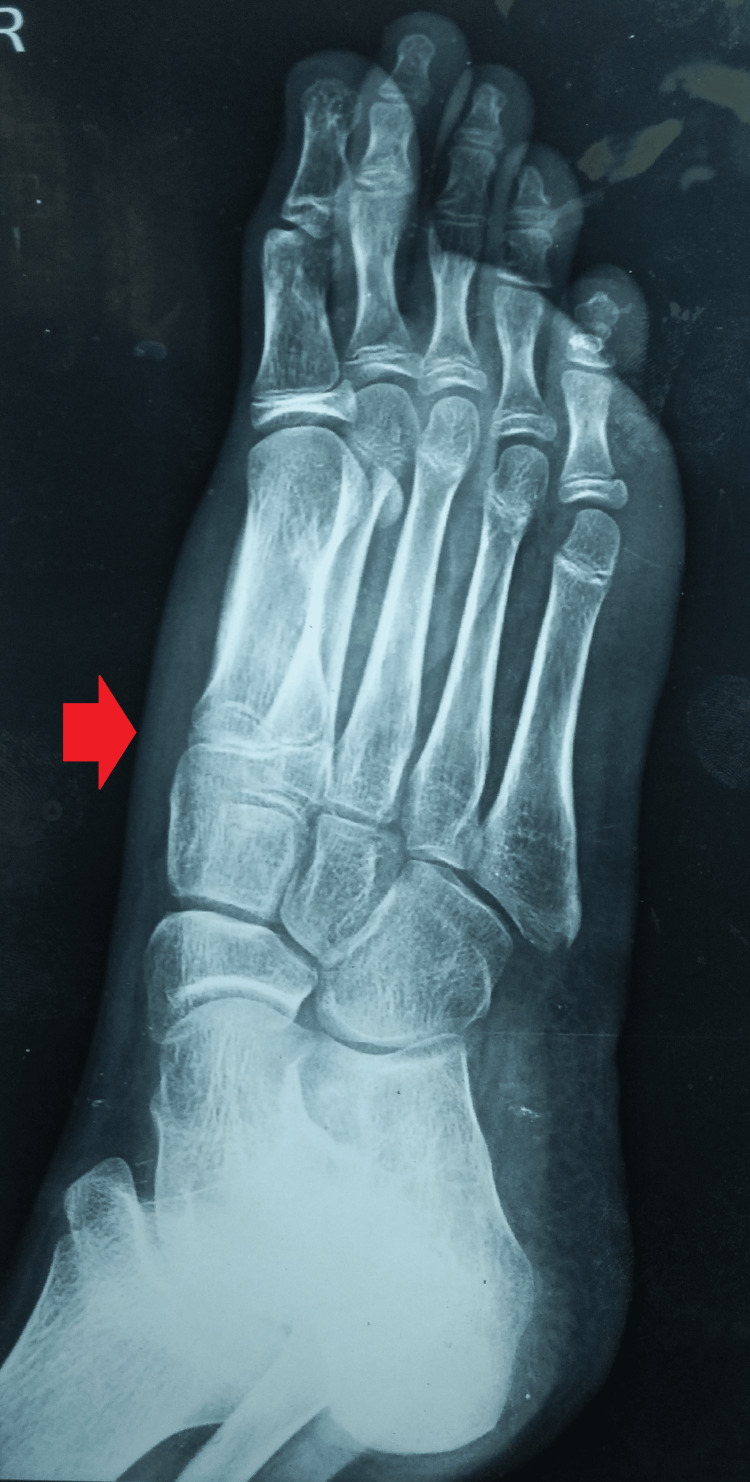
Plain radiograph oblique view of the right foot showing soft tissue swelling and involvement of the first metatarsal head
